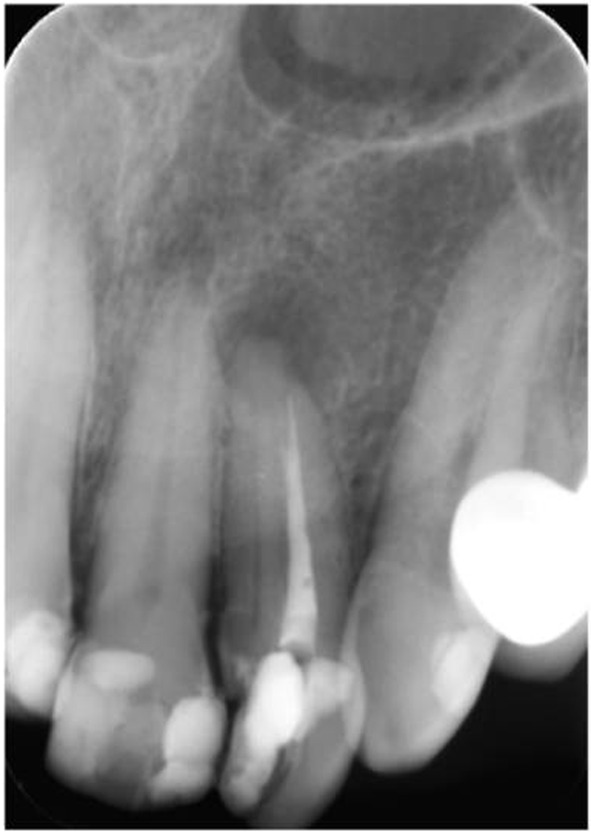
Preoperative periapical radiography.
